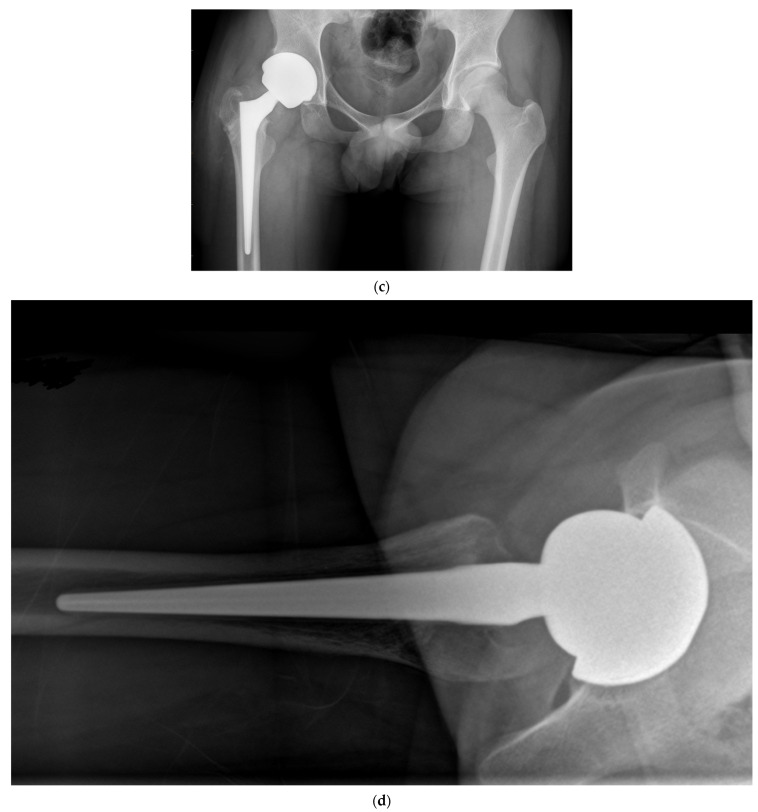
(a) Anteroposterior pelvis radiograph of 19-year-old men with right femoral head avascular necrosis following a slipped capital femoral epiphysis treated with canulated screws. During preoperative templating, acetabular, and femoral components sizes were estimated to be small. (b) Preoperative lateral view of the right hip. (c) Postoperative anteroposterior pelvis radiograph showing a CoC LDH THA with a 13 mm conical stem and a 46 mm monobloc ceramic acetabular component with a bearing diameter of 36 mm. (d) Postoperative lateral view of the right hip replacement.
